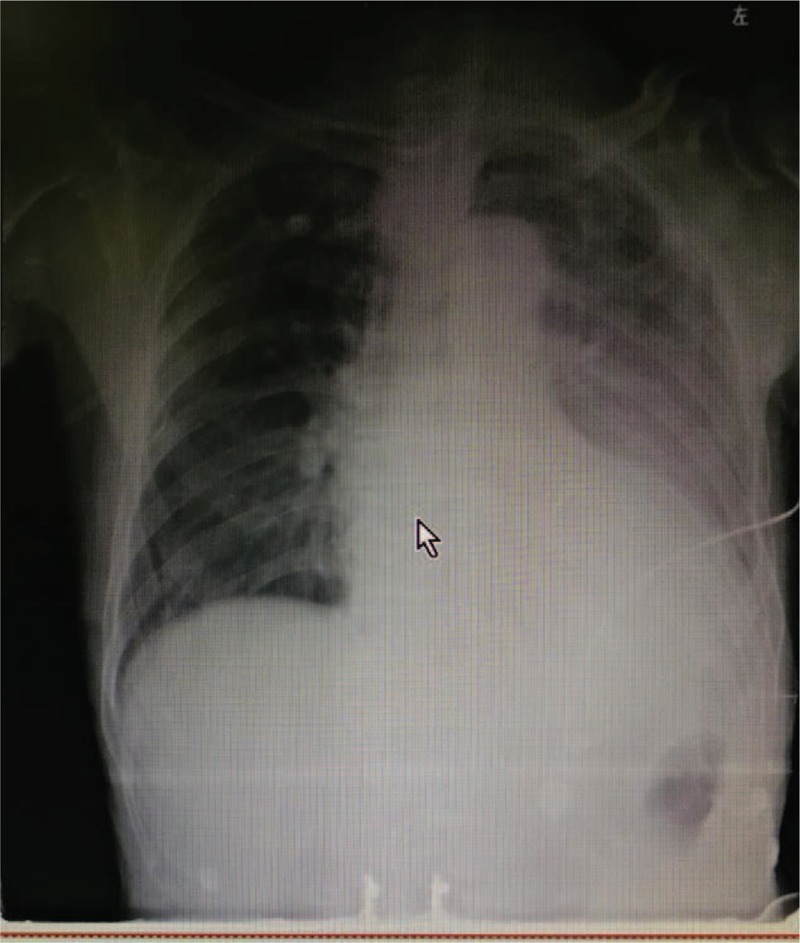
Chest X-ray at admission of ICU showed pulmonary infection with an enlarged heart. ICU = intensive care unit.
